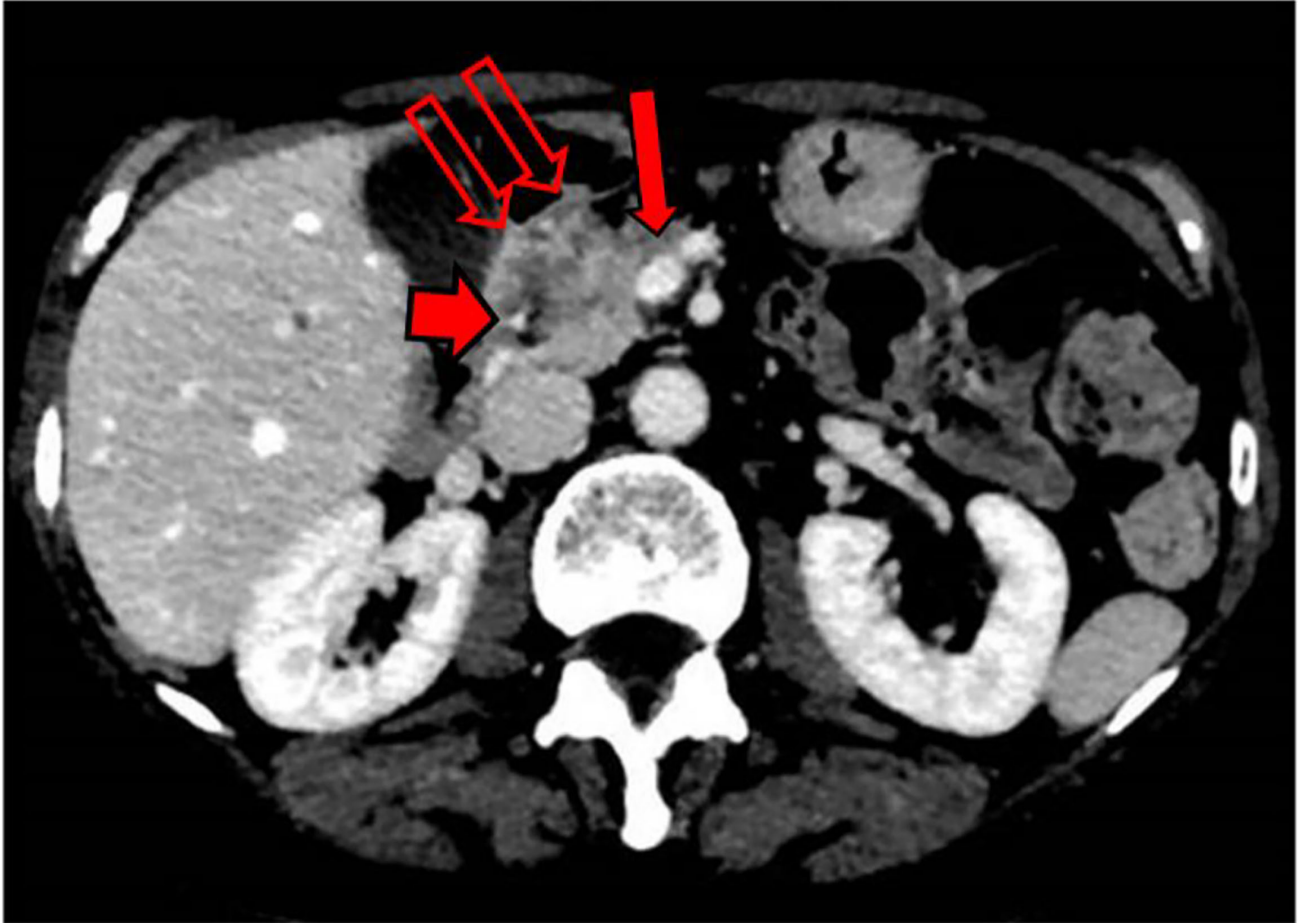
Abdomen CT scan. Single thin arrow, the head pancreatic tumor; short broad arrow, tumor involvement of the superior duodenal-pancreatic artery; twin open arrows, tumor involvement of the portal vein near to the spleno-portal axis.
